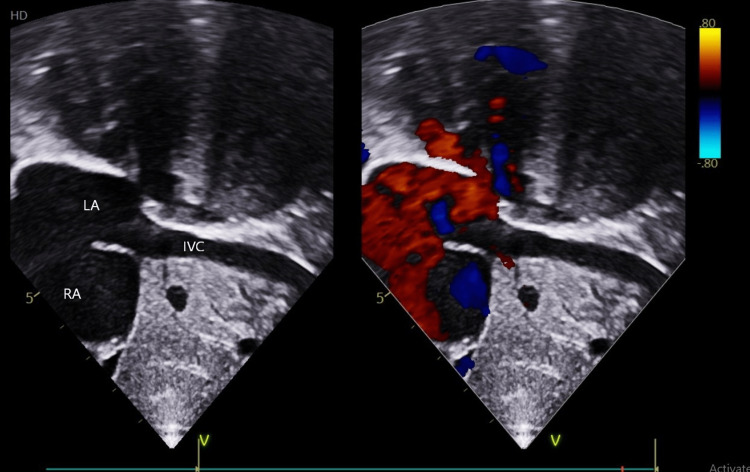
Case 1 - Echocardiography showing IVC draining into the LA. IVC: inferior vena cava; A: left atrium; RA: right atrium
